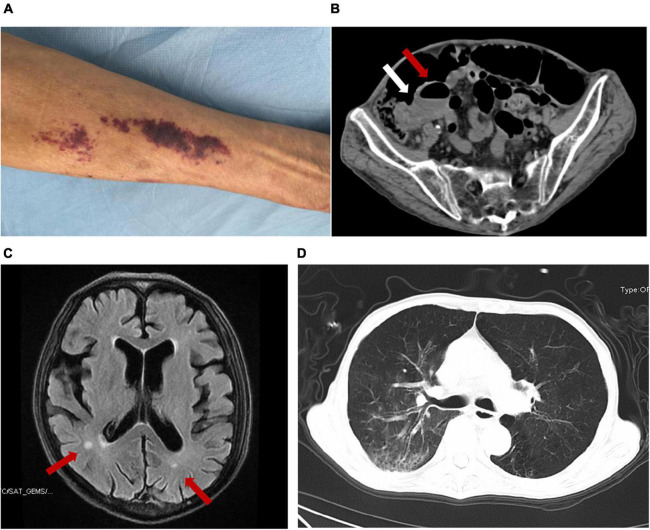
The presentation of this case and the detection of *S. stercoralis* larvae. Anaphylactoid purpura were present on left upper limb (A). Abdominal CT imaging showed that the ileocecum was swollen and thickened (white arrow) and the proximal small intestine was obstructed with air-fluid level (red arrow) (B). Brain MRI showed patches near bilateral ventricles (red arrow) and partial endocranium thickened (C). Chest CT revealed bronchiectasis with secondary pulmonary infection (D).
